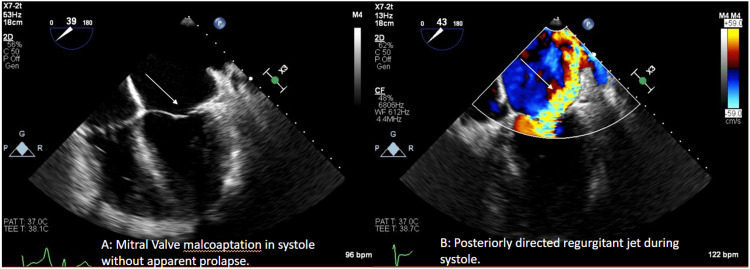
Preoperative Transesophageal Echocardiogram demonstrating severe Mitral Regurgitation due to systolic restriction of the anterior leaflet (A) with a posterior regurgitant jet (B). White arrows indicate findings noted.
